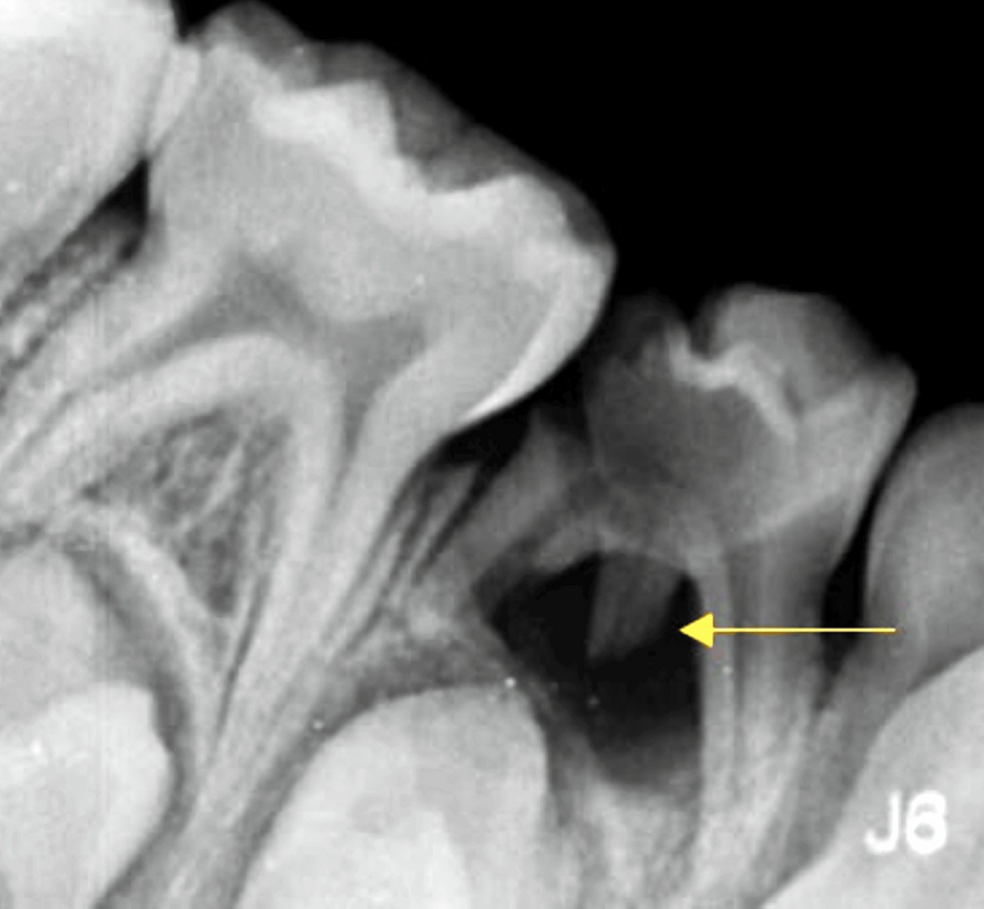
Depiction of the preoperative radiograph of RE of the left deciduous mandibular first molar RE: Radix entomolaris
